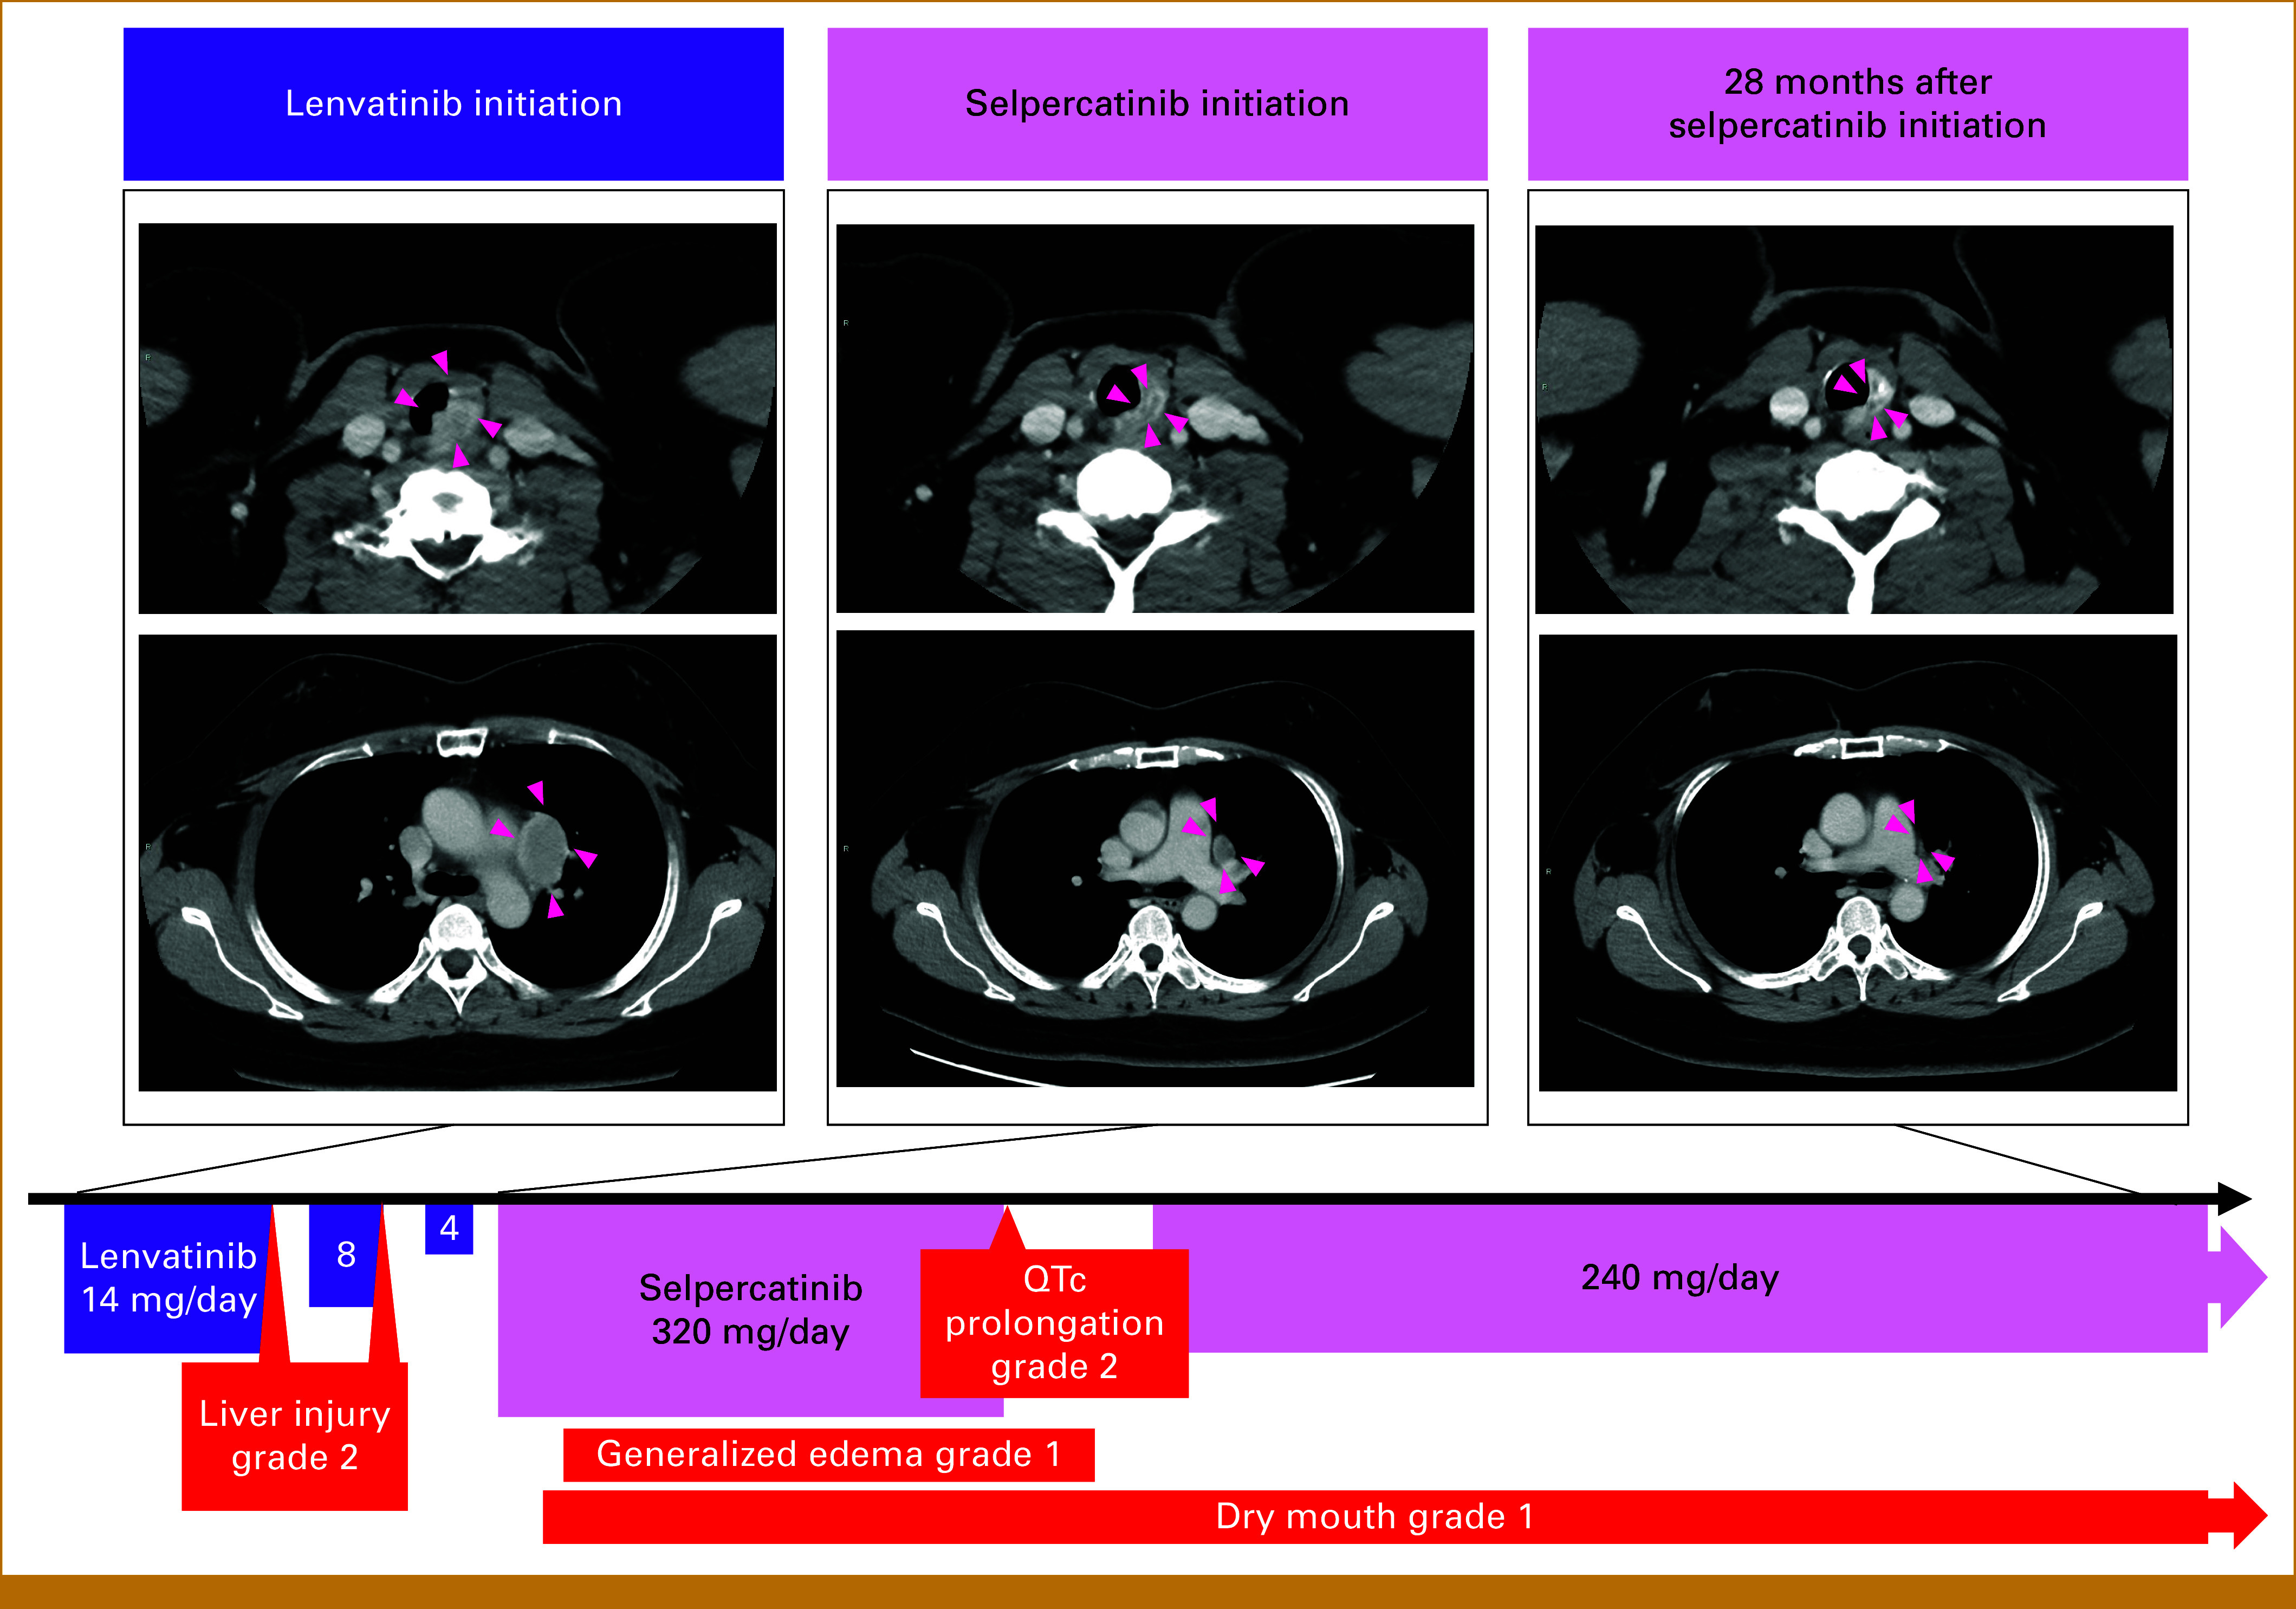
Radiologic response to selpercatinib. Representative computed tomography images show shrinkage of the neck and mediastinal lesions after selpercatinib initiation. The timeline summarizes systemic therapies, genomic assays, and adverse events.
